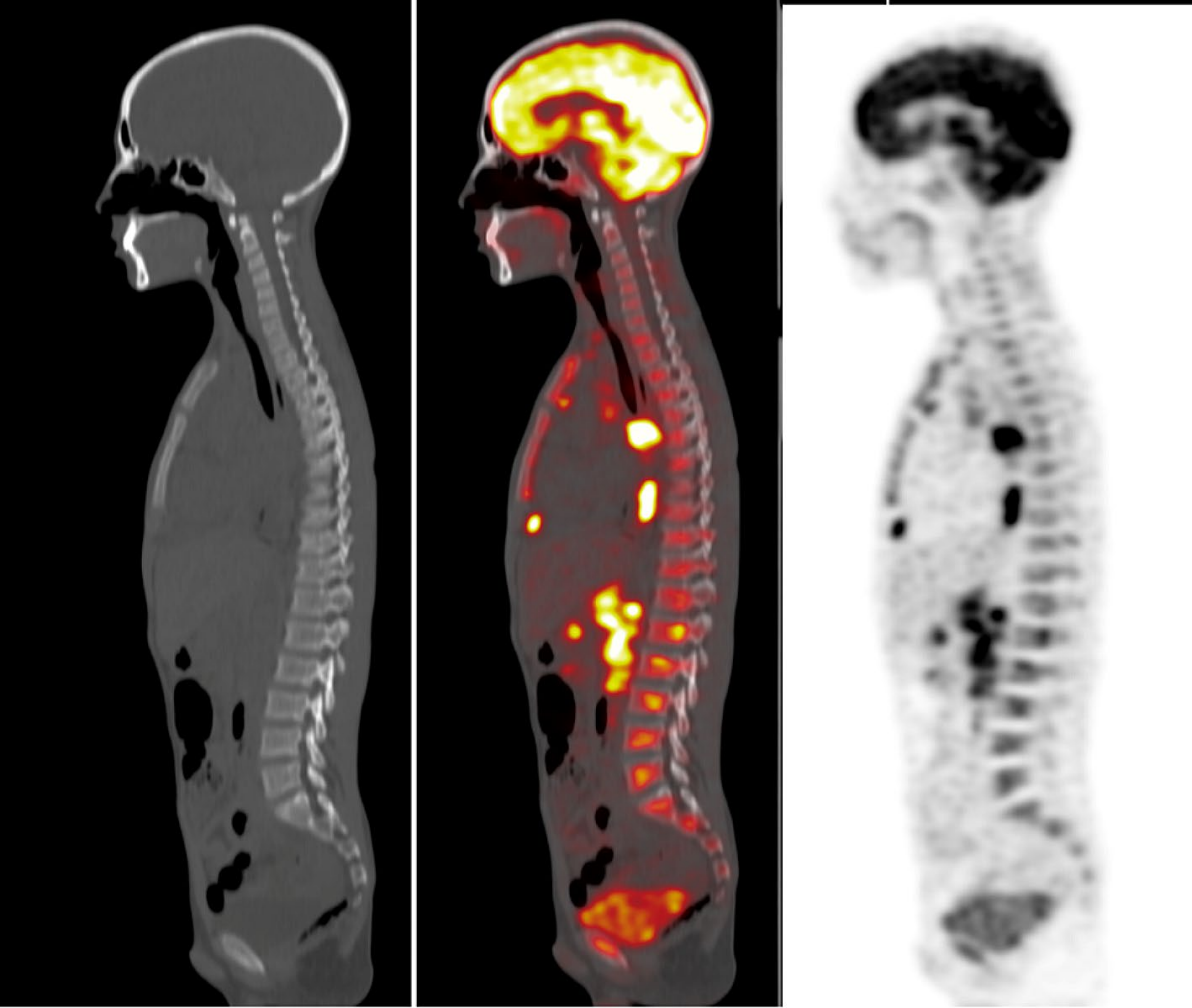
A 14-year-old male with Hodgkin’s lymphoma (nodular sclerosing subtype) PET, fused PET/ CT images and CT images in sagittal view showing focal BM uptake without corresponding CT changes. BMB was negative
